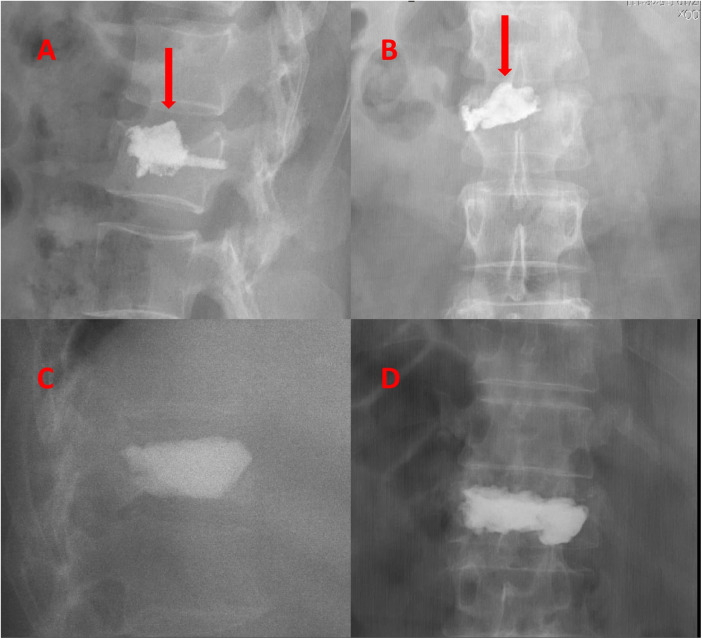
(A,B) Postoperative anteroposterior and lateral x-ray of the vertebral body showing cement leakage into the intervertebral space, with the patient reporting early postoperative low back pain. (C,D) Postoperative anteroposterior and lateral x-ray of the vertebral body showing good distribution and positioning of the bone cement, with the patient reporting the disappearance of pain symptoms.
